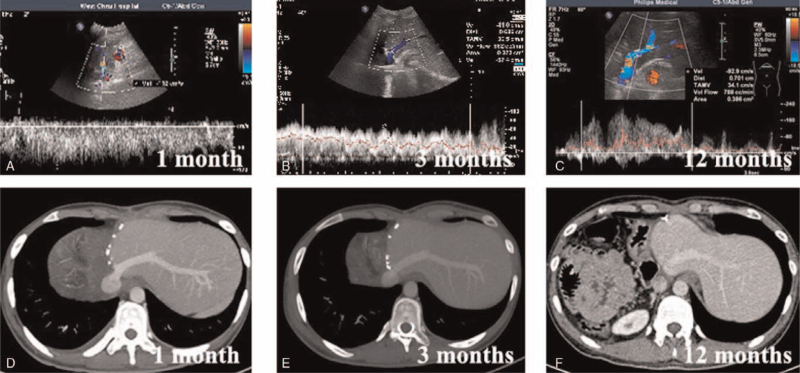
Results of postoperative CT scans and color Doppler ultrasonography.
